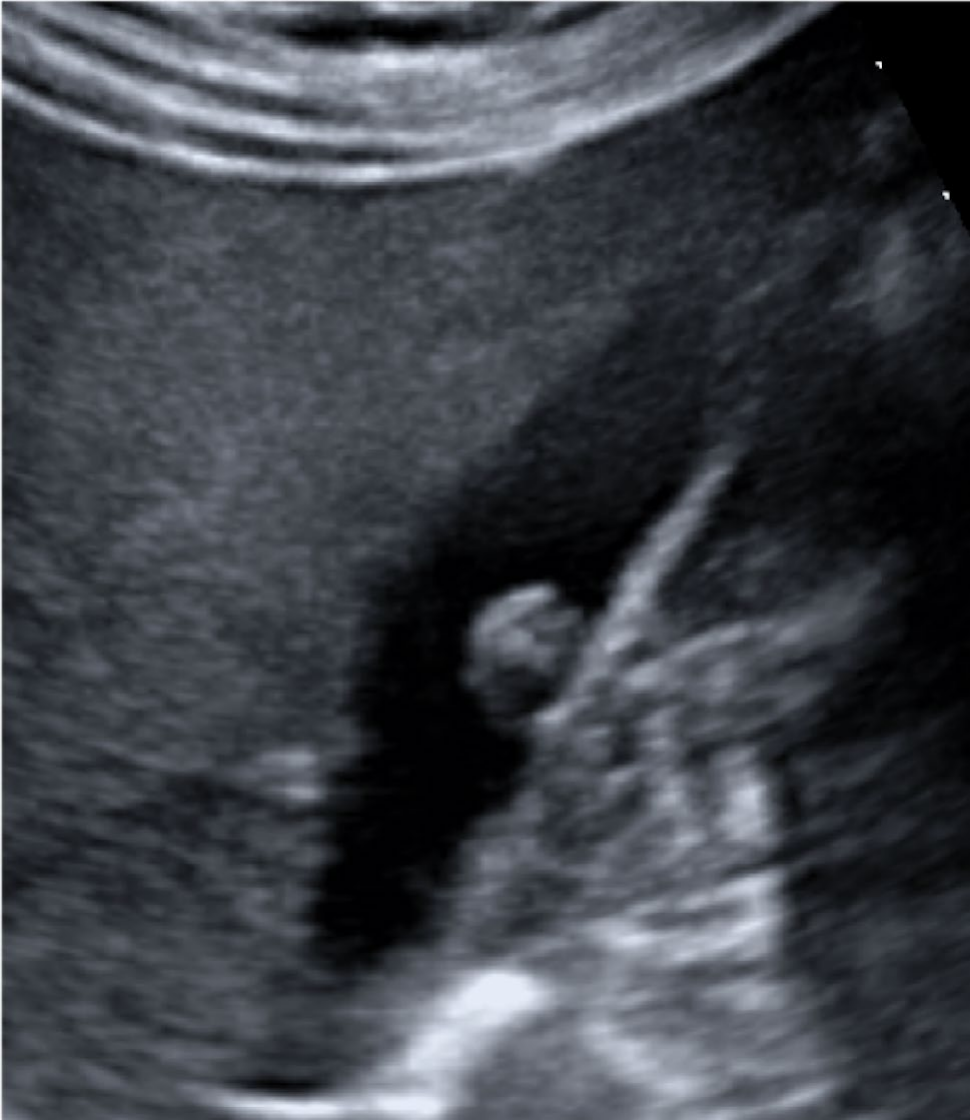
The ultrasound image of the adenoma of the gallbladder appears as a singular homogeneous polyp, isoechoic with the liver parenchyma and sessile
